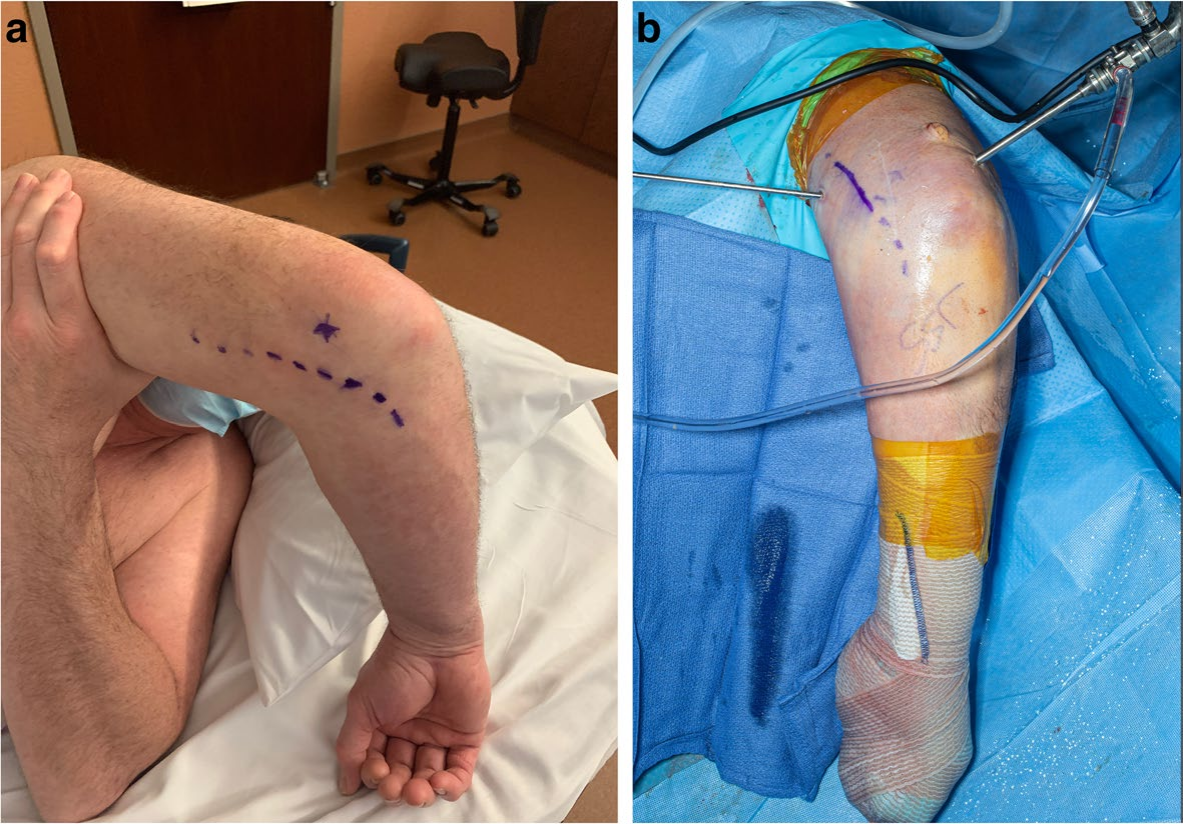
Ultrasound tracing of the course of a transposed ulnar nerve may allow safe arthroscopic osteocapsular arthroplasty. A Marking of the ulnar nerve course prior to arthroscopy. B Arthroscopic portals avoiding the location of the previously transposed ulnar nerve
